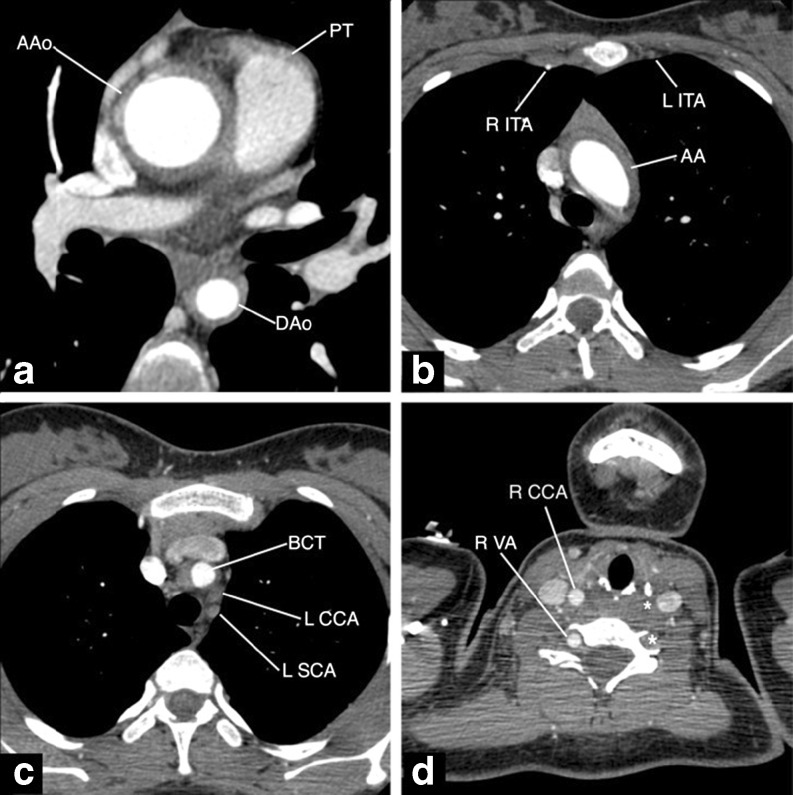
Spiral computed tomography angiography examination, cross-section slices presenting circumferential thickening of aortic wall. a Level of AAo and DAo with slightly thickened wall of proximal section of pulmonary trunk; b level of aortic arch with nonenhanced L ITA; c proximal sections of aortic arch branches with occluded L CCA and L SCA; d widening of R VA, lack of opacification of L VA and L CCA (marked with *arrows*). *AAo* ascending aorta, *AA* aortic arch, *DAo* descending aorta, *BCT* brachiocephalic trunk, *CCA* common carotid artery (*L* left, *R* right), *SCA* subclavian artery (*L* left, *R* right), *VA* vertebral artery (*L* left, *R* right), *ITA* internal thoracic artery (*L* left, *R* right), *PT* pulmonary trunk
